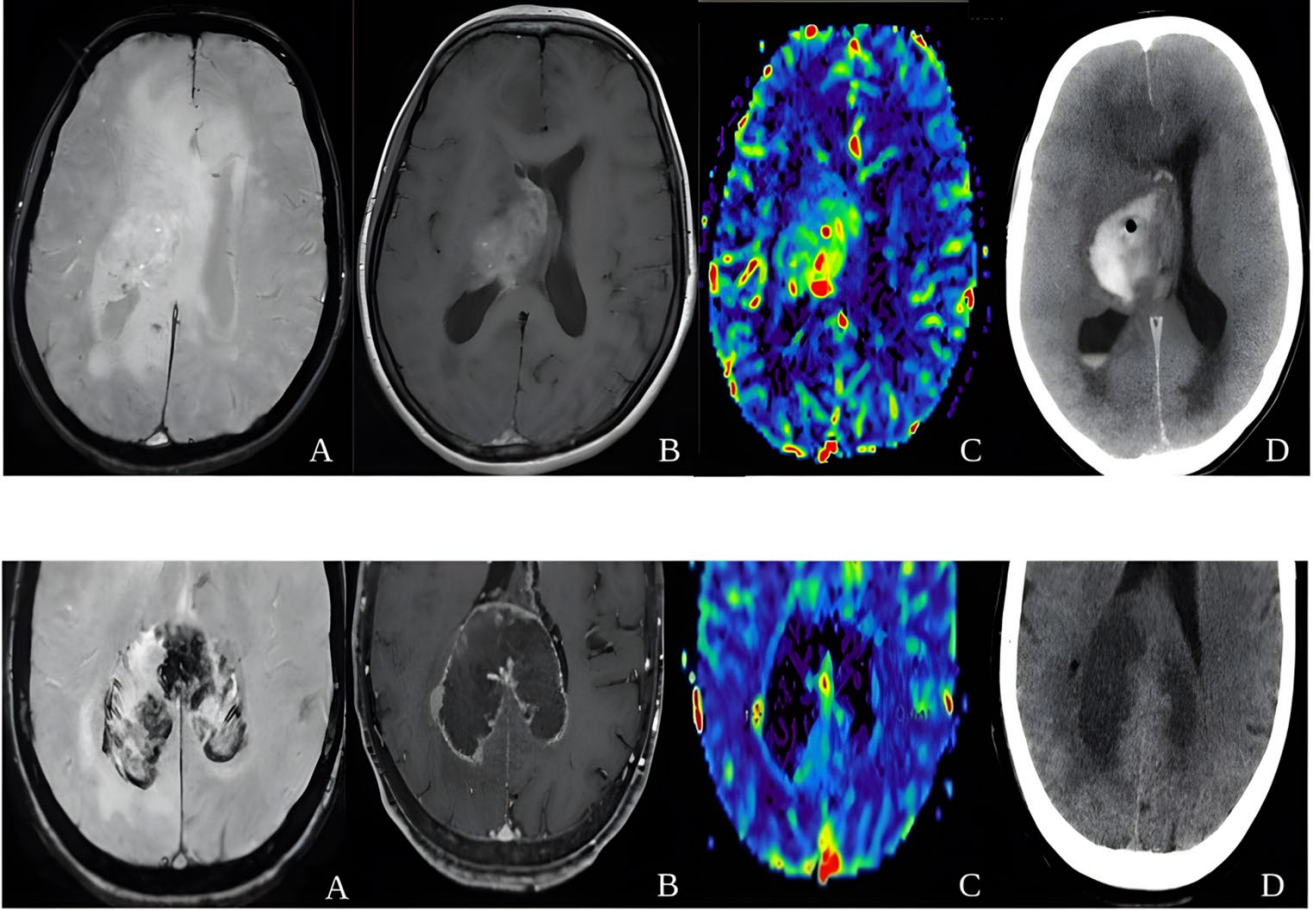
Upper row: (A) SWI; (B) T1w FSE post-Gad; (C) DSC-PWI CBV map; (D) CT post-biopsy. Deep-seated GB with ITSS score = 0 (A) and large post-biopsy hemorrhage (D). Lower row: (E) SWI; (F) 3D-T1w FSPGR post-Gad; (G) DSC-PWI CBV map; (H) CT post-biopsy. Butterfly GB in the splenium of corpus callosum (sWM), with ITSS score = 3 (E), some hotspot of high rCBV (G), without hemorrhage after biopsy (H)
